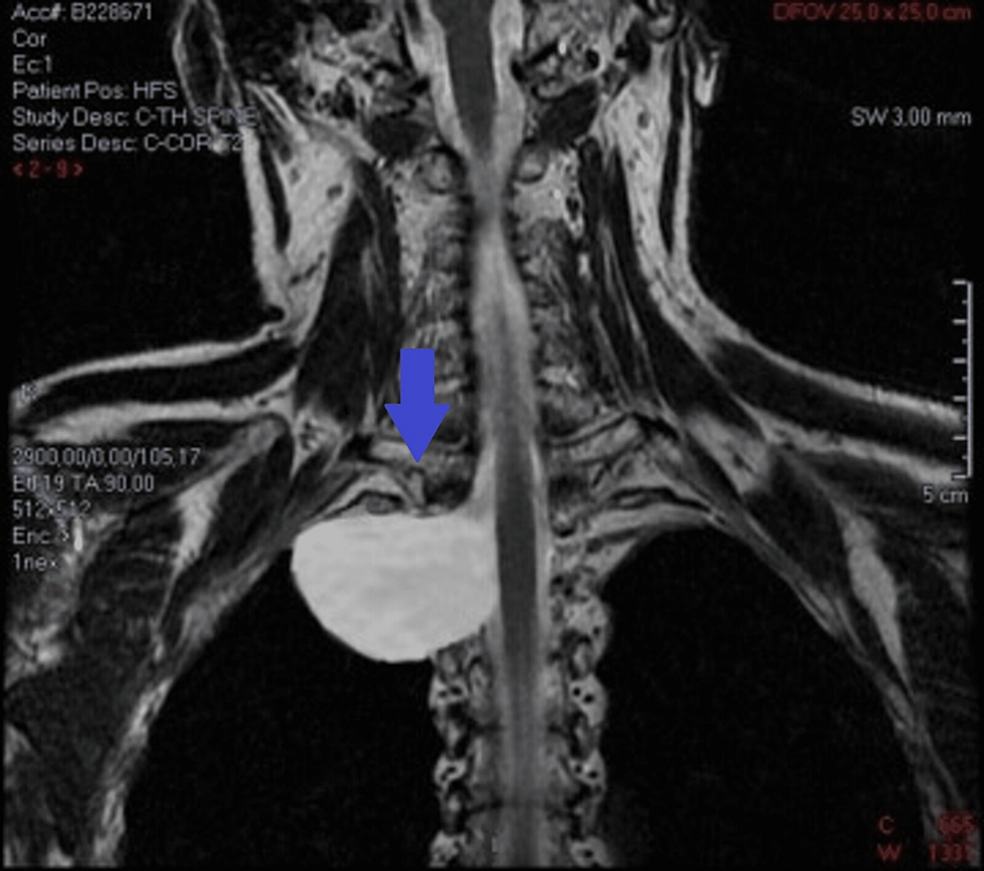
MRI showing the intrathoracic meningocele (blue arrow)
